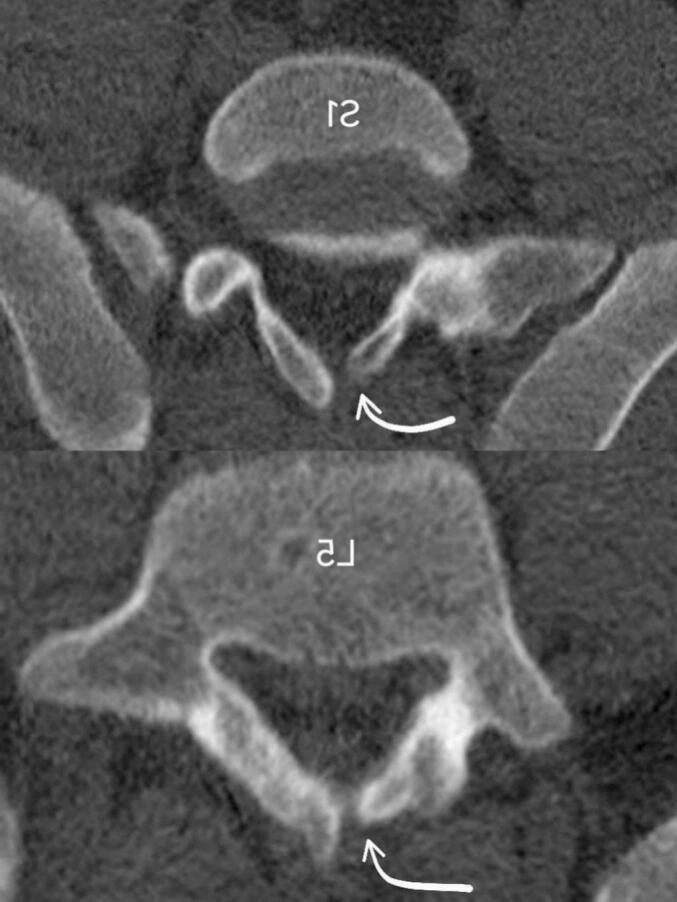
Axial CT scan of lumbosacral region, white arrows show a bone defect in L5 and S1 lamina with sclerotic margins.
